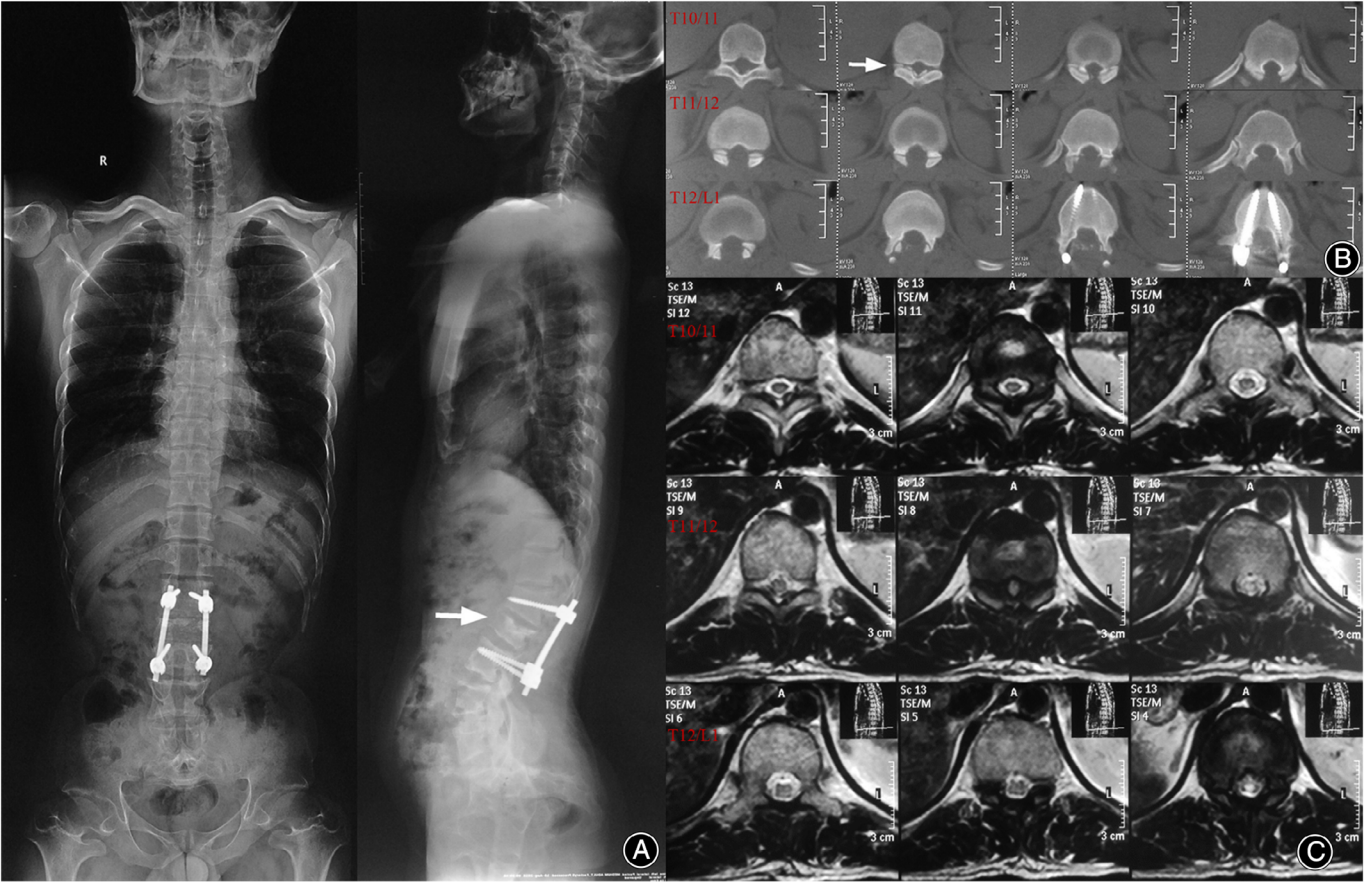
Radiographic data prior to reoperation in case 1. Lateral *x*‐ray image shows the wedge‐shaped changed L2 vertebrae (arrow in A). Axial CT shows ossification of the ligamentum flavum at the level of T10–11 (arrow in B).
